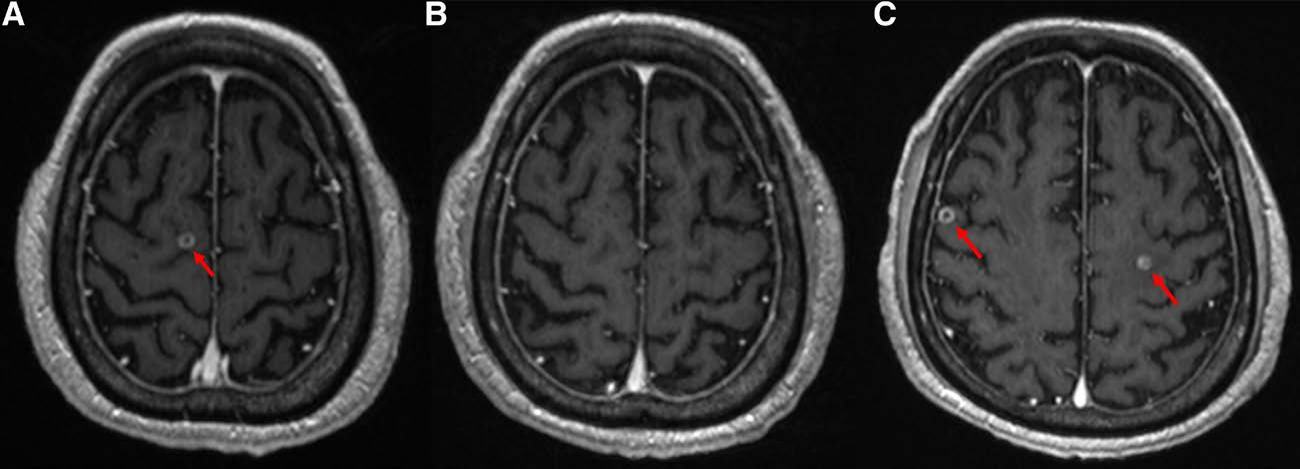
Changes in the brain MRI of the patient. (A) Baseline before WBRT on pemetrexed/cisplatin chemotherapy; (B) after WBRT: complete response status in brain; and (C) progressive disease status. MRI = magnetic resonance imaging, WBRT = whole-brain radiation therapy.
